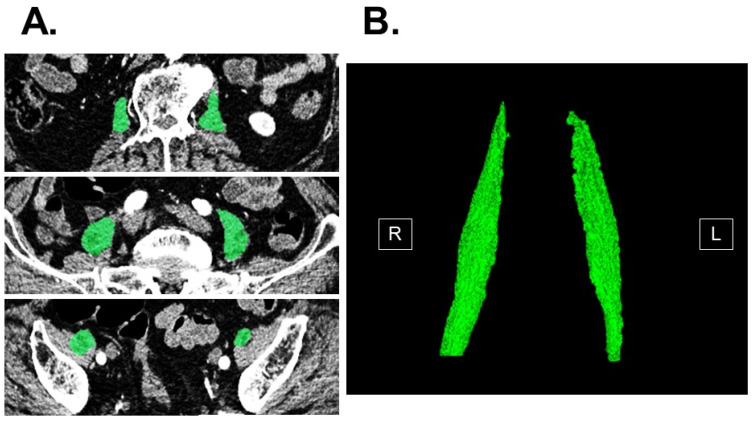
Measurement of total psoas muscle mass volume using abdominal computed tomography imaging. Bilateral psoas muscles (green) in horizontal section were automatically traced by the software in each slice (A). All slices were combined and three-dimensional image of bilateral psoas muscle with calculated volume was obtained (B).
